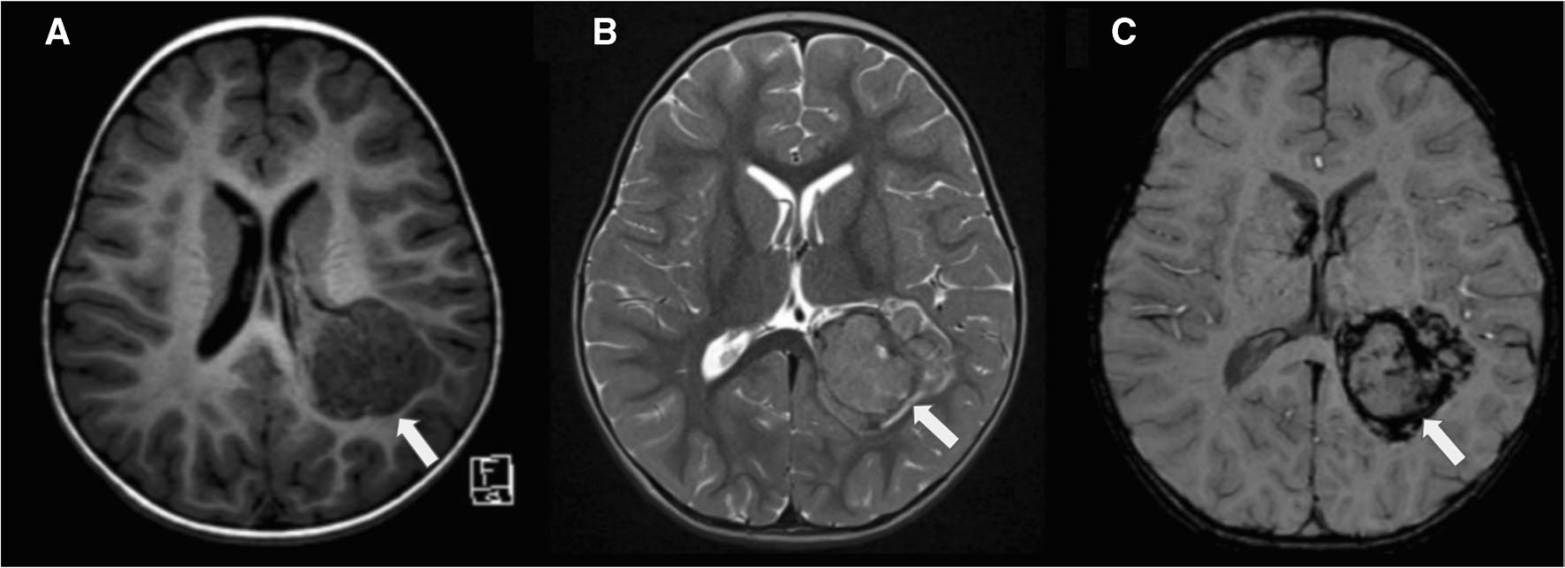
Choroid plexus carcinoma (whiter arrows) in a 24-month-old boy with headaches and recent seizures. A An axial T1-weighted image shows a macrolobulated, hypointense mass with signs of brain invasion and no hydrocephalus. B An axial T2-weighted image reveals that the lesion is predominantly isointense, with minimal surrounding CSF. In addition, there are signs of brain invasion and a mild degree of adjacent vasogenic edema. C An axial SWI image shows hypointense material, mainly at the periphery of the masses, consistent with haemorrhage
